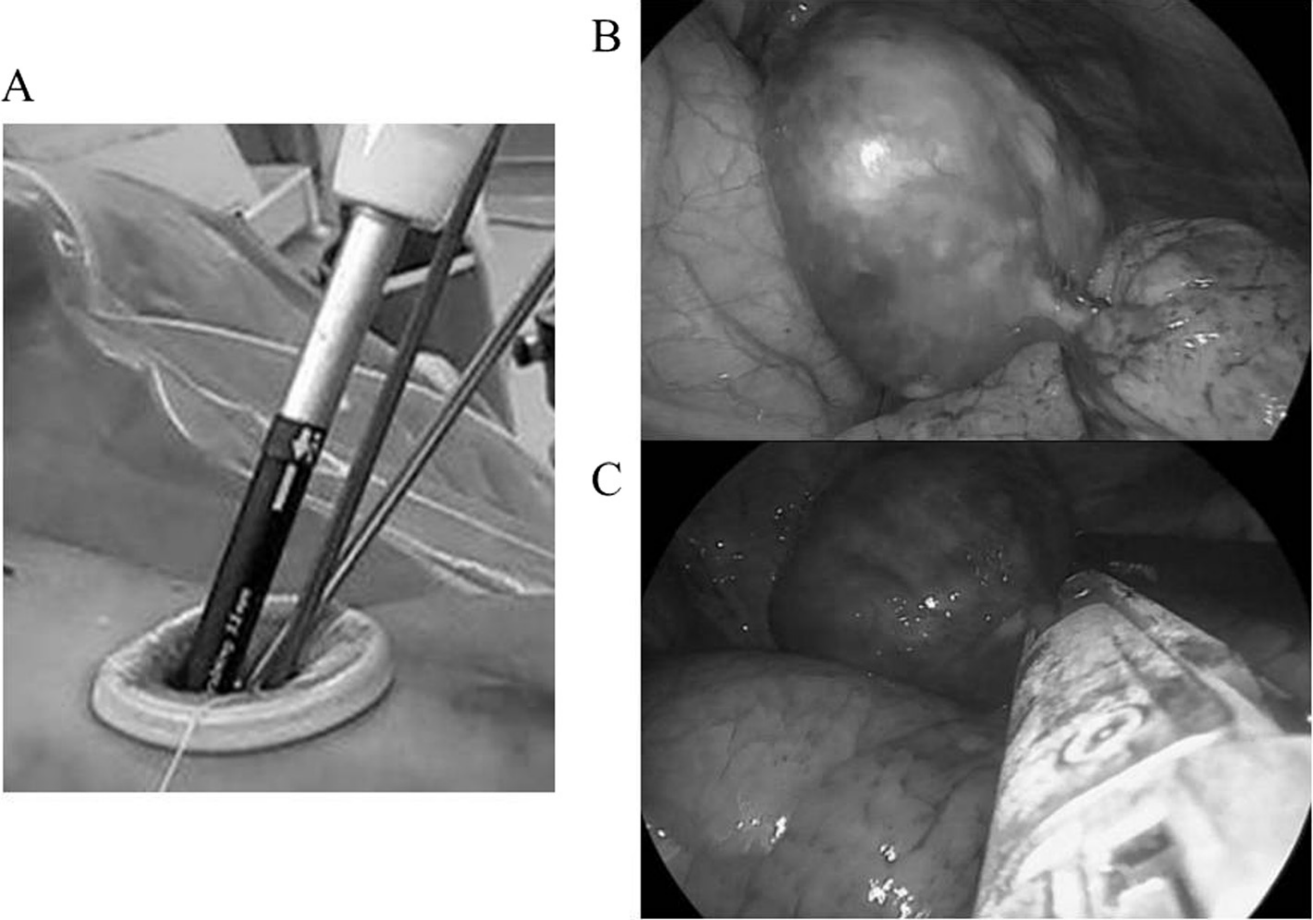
Intraoperative findings. (A) A 2.5 cm skin incision was made, and a wound retraction system (Alexis Wound Retractor, Applied Medical, Rancho Santa Margarita, CA USA) was placed through the incision. (B) A pedunculated tumor protruded into the thoracic cavity from the visceral pleura, and was attached to the left upper lobe. (C) The tumor stalk was resected using an articulating endostapler (Covidien, Norwalk, CT, USA).
